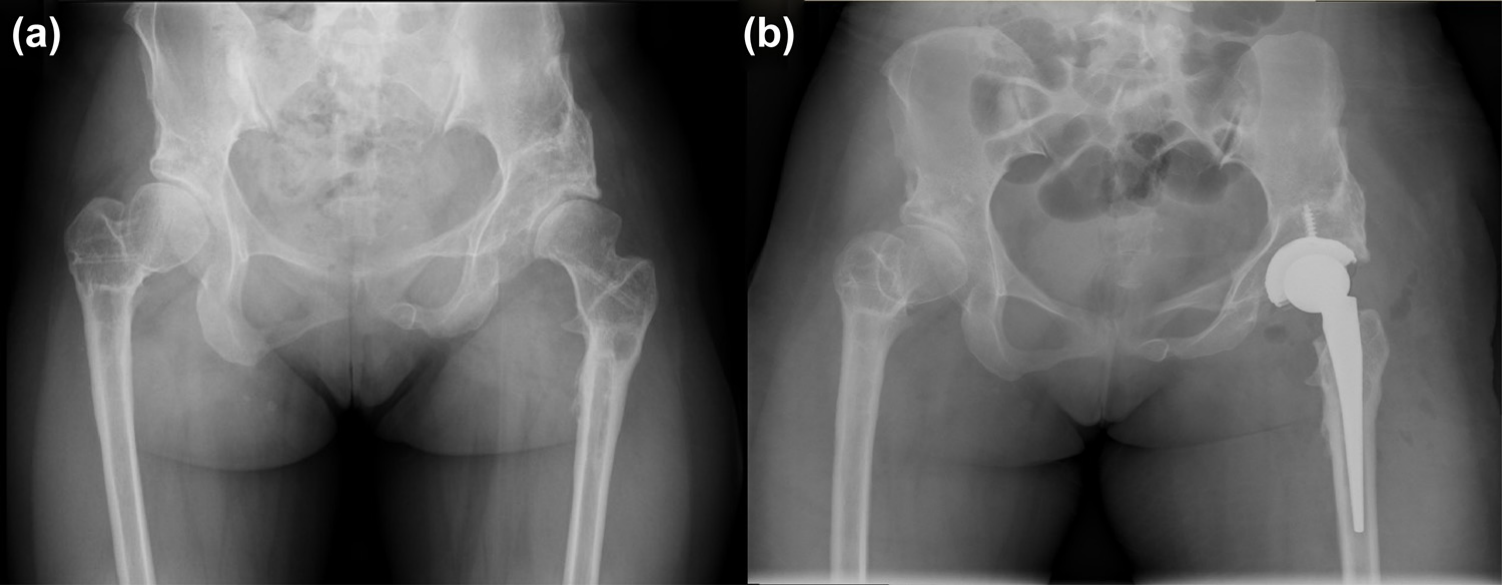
The X-rays of the case. (a) Preoperative plain radiography of the pelvis. (b) Postoperative plain radiography of the pelvis.
